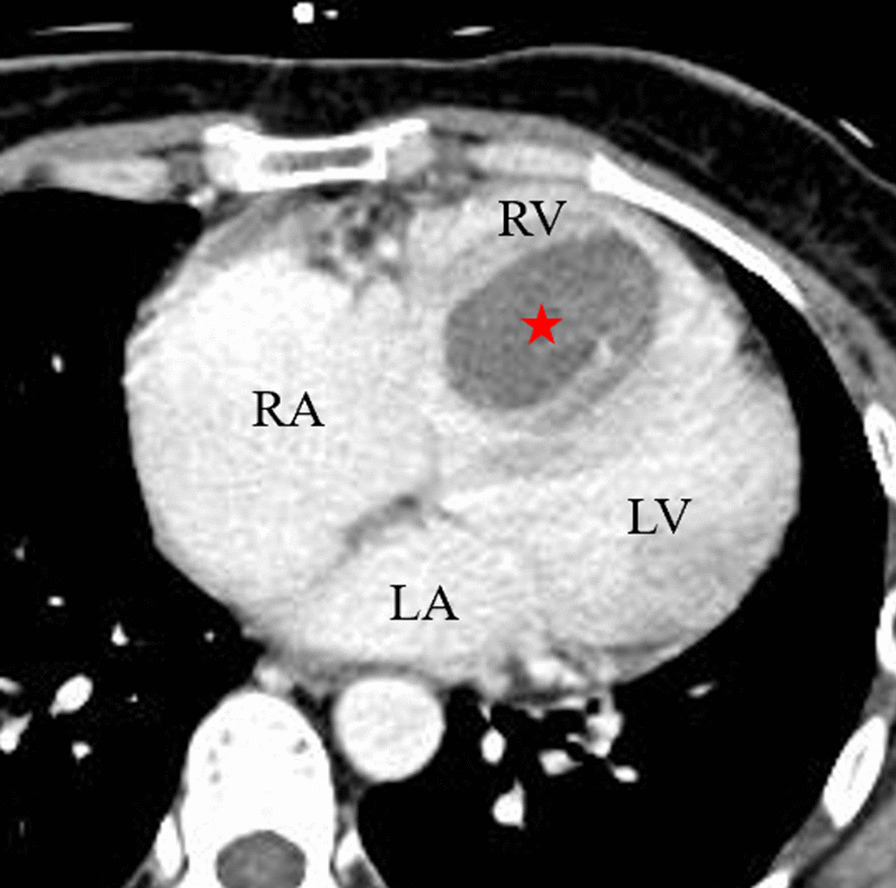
Contrast-enhanced computed tomography showed the tumor in RV had no contrast effect (red star); *LA* left atria, *LV* left ventricle, *RA* right atria, *RV* right ventricle
